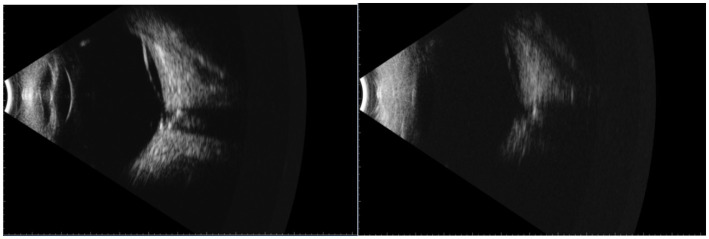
Ocular B-scan ultrasound (ACCUTOME A Halma company diagnostic ultrasound, USA, probe frequency 15 MHz) indicated: In the right eye, an arched white reflective band (retina) was seen floating within the vitreous cavity. Behind this band was an echo-free dark area. One end of the band connected to the optic disc. The optic disc region showed a localised, well-defined, regularly shaped depression communicating with the vitreous cavity. Its deep aspect extended posteriorly toward the optic nerve axis, forming a funnel-shaped dark area in the posterior pole. The lesion exhibited a slightly inverted “bottleneck” configuration. The optic disc appeared slightly enlarged and depressed, with irregular hypoechoic signals within the depression.
